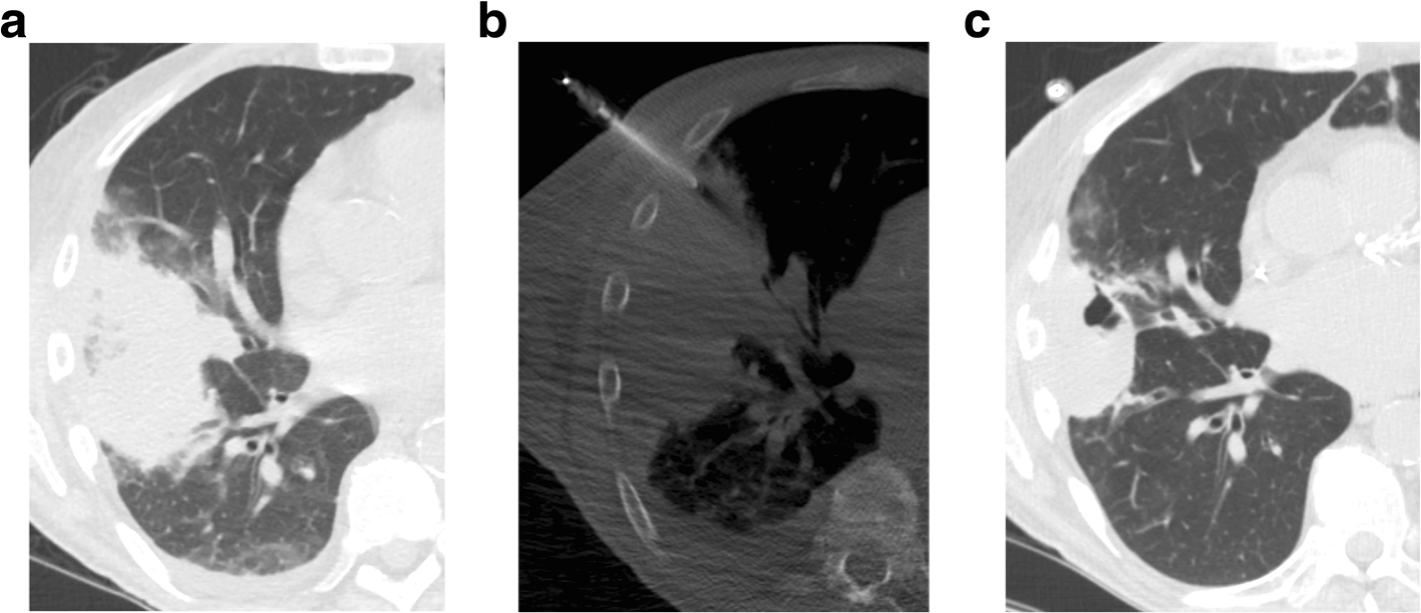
a) Axial CT scan in a 69-year-old man status post kidney transplant demonstrates an area of consolidation and surrounding ground glass opacity in the right middle and lower lobe. He underwent bronchoalveolar lavage and transbronchial biopsy which found acute hemorrhage and hemosiderosis. b) Axial CT scan obtained during PTNB, performed 2 days after bronchoscopy, shows the needle within the consolidation in the right middle lobe. Fungal hyphae were identified on cytologic evaluation and aspergillus was identified on accompanying microbiology culture. c) Axial CT performed three months later, on treatment with ambisome, shows the area of consolidation improving
